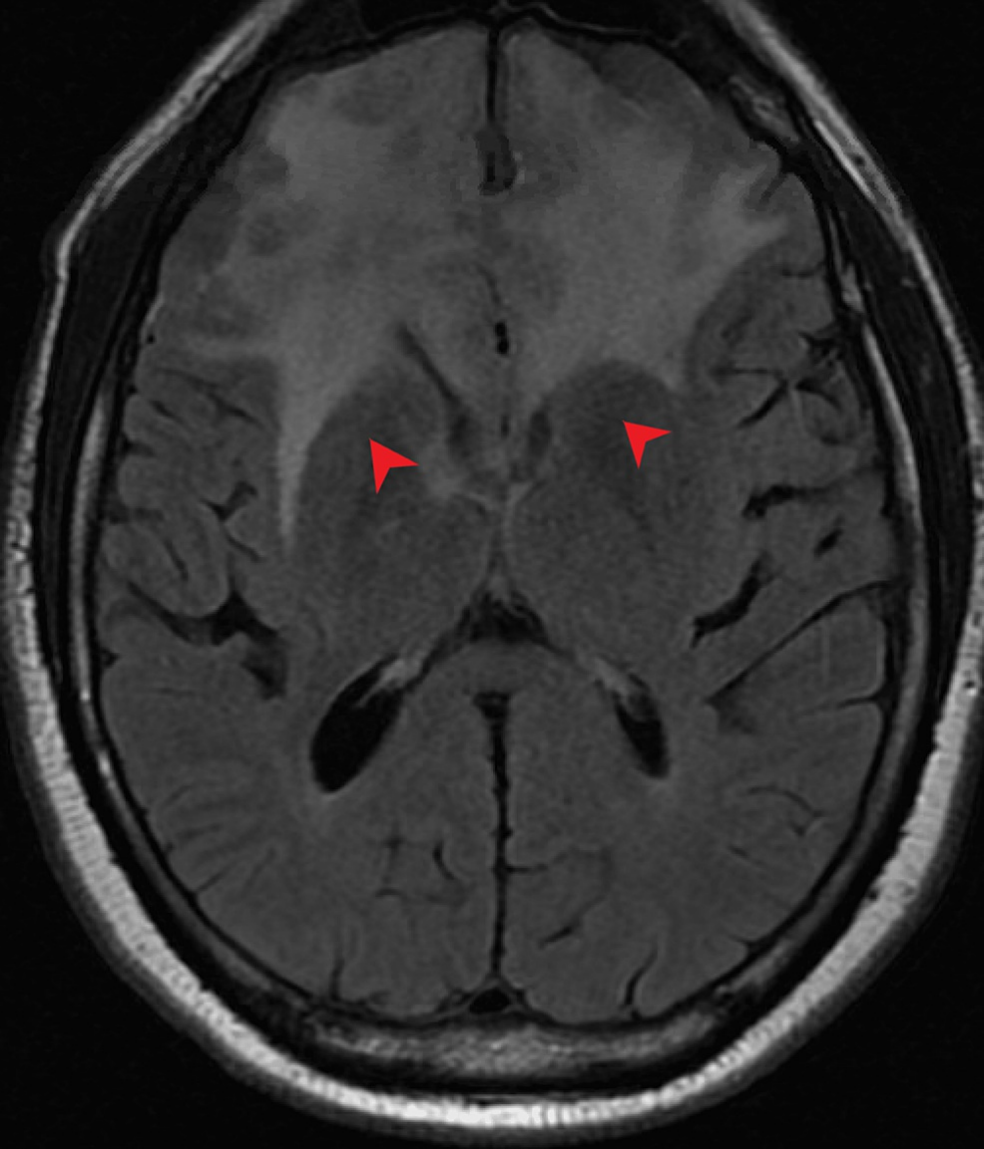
Axial T1-weighted MRI of the brain with contrast revealing extensive nodular leptomeningeal enhancement along the bilateral frontal lobes, suprasellar cistern, right Sylvian fissure, and right basal ganglia with associated extensive vasogenic edema extending to the lateral ventricles (red arrows)
